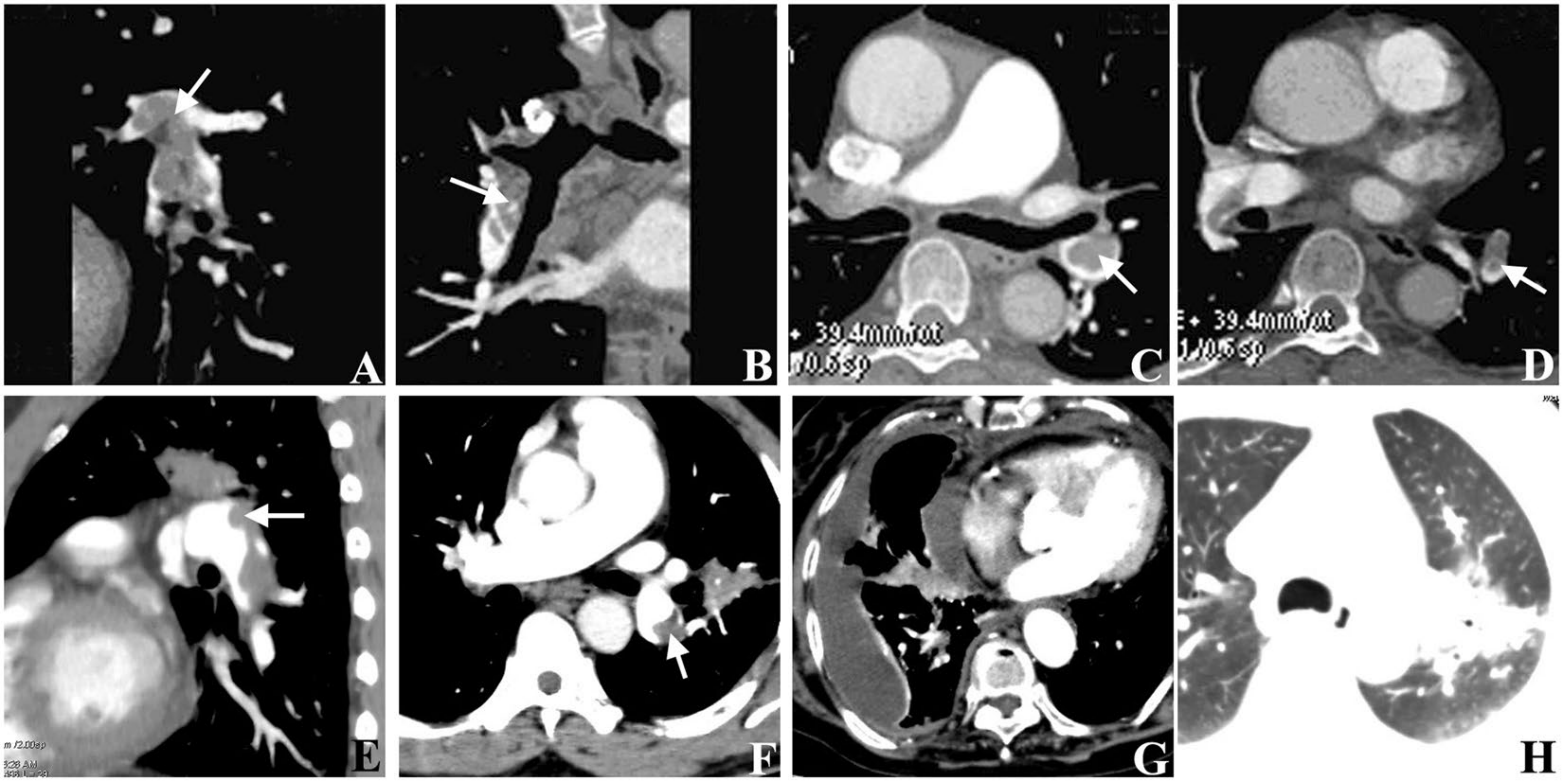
CT manifestations of pulmonary embolism. (A,B) The sagittal (A) and coronal (B) MPR images showed the right inferior pulmonary artery were filled with a filling defect, which belonged to a complete occlusion type of pulmonary embolism. (C,D) The original transverse axis images showed the central filling defect surrounded by contrast media in the left inferior pulmonary artery, which belonged to a central type of pulmonary embolism. (E,F) The coronal (E) and axis images (F) showed the filling defects in one side of the artery, which belonged to a peripheral type. (G) The pleural effusion and pericardial effusion was showed in one patient. (H) The pulmonary infarction was showed in one patient. Arrows indicate the embolus.
